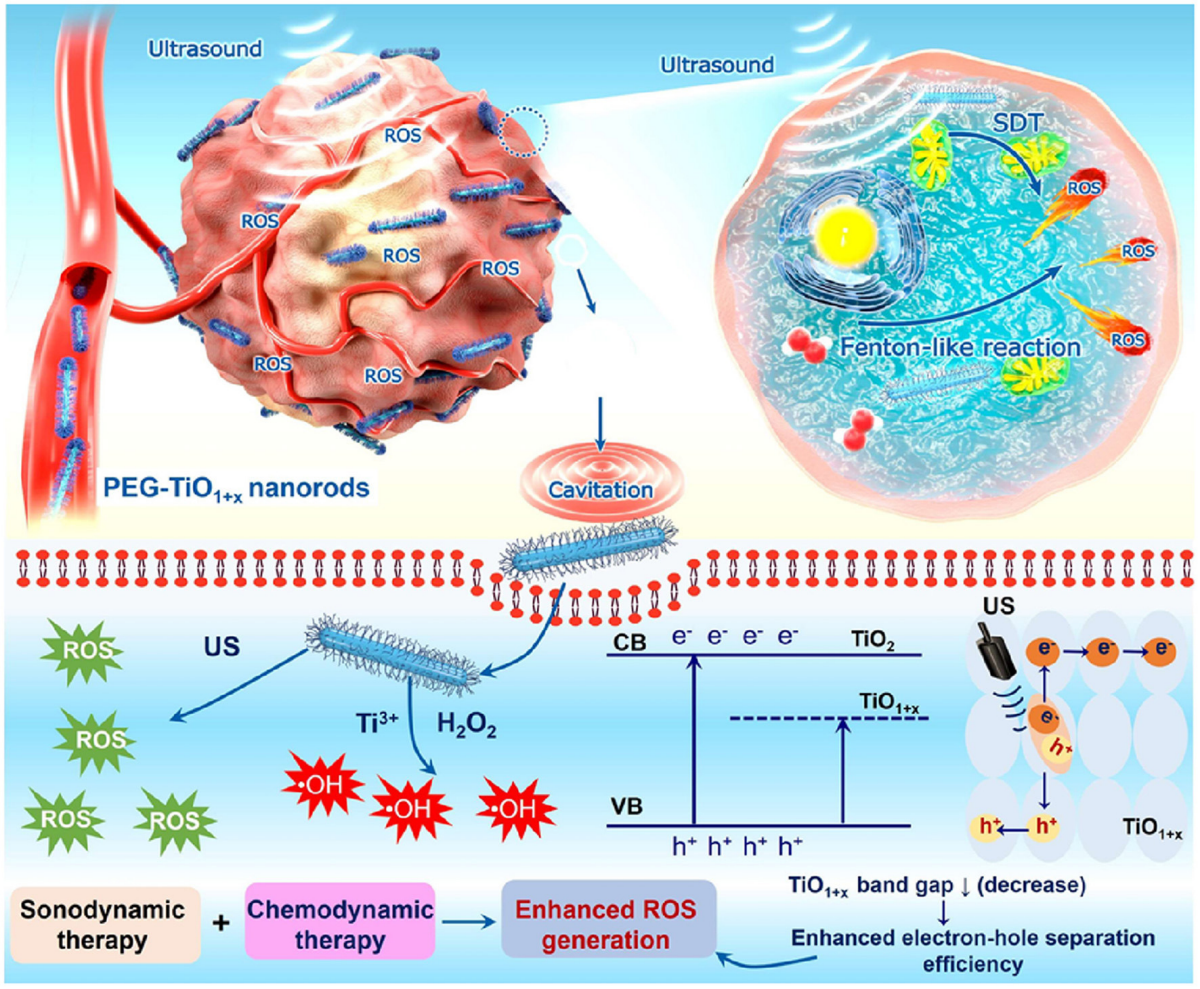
Application of PEGylated titanium monoxide nanorods (TiO_2_ NRs) in sonodynamic therapy. Oxygen-deficient TiO_2_ NRs have shown higher reactive oxygen species (ROS) generation upon ultrasound irradiation. Reproduced with permission (Wang et al., 2020d) *Copyright* © *2020, American Chemical Society*.
